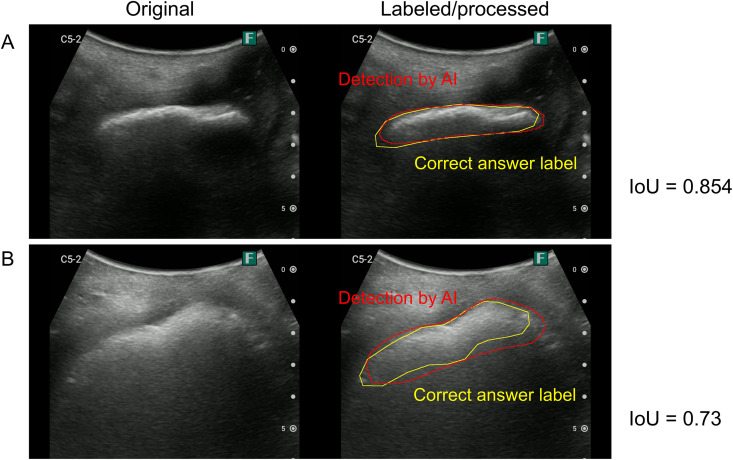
Example of ultrasound image determined as true positive. (Left) Original image. (Right) Areas labeled as correct by the sonographer (yellow) and detected by AI (red). (A) IoU = 0.584, (B) IoU = 0.73, and IoU > 0.5, resulting in a true positive decision. AI, artificial intelligence; IoU, intersection over union.
